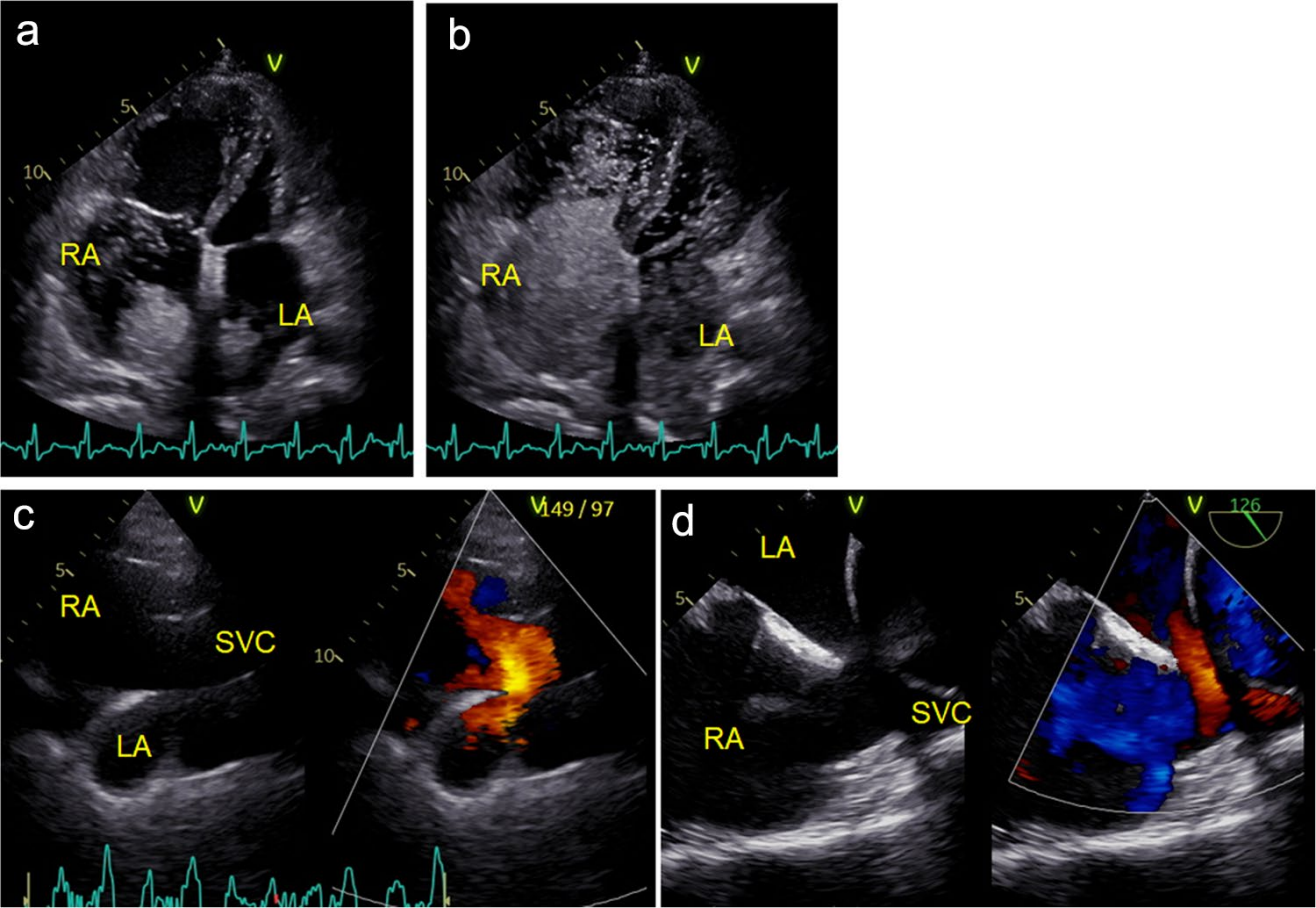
A case of superior sinus venosus-type ASD. a, b Apical four-chamber view of transthoracic echocardiography with agitated saline contrast. Agitated saline contrast injected from the right upper extremity appears and spreads simultaneously in the bilateral atria. c Visualization of the defect from the right sternal window. d Transesophageal echocardiographic image. *ASD* atrial septal defect, *LA* left atrium, *RA* right atrium, *SVC* superior vena cava
